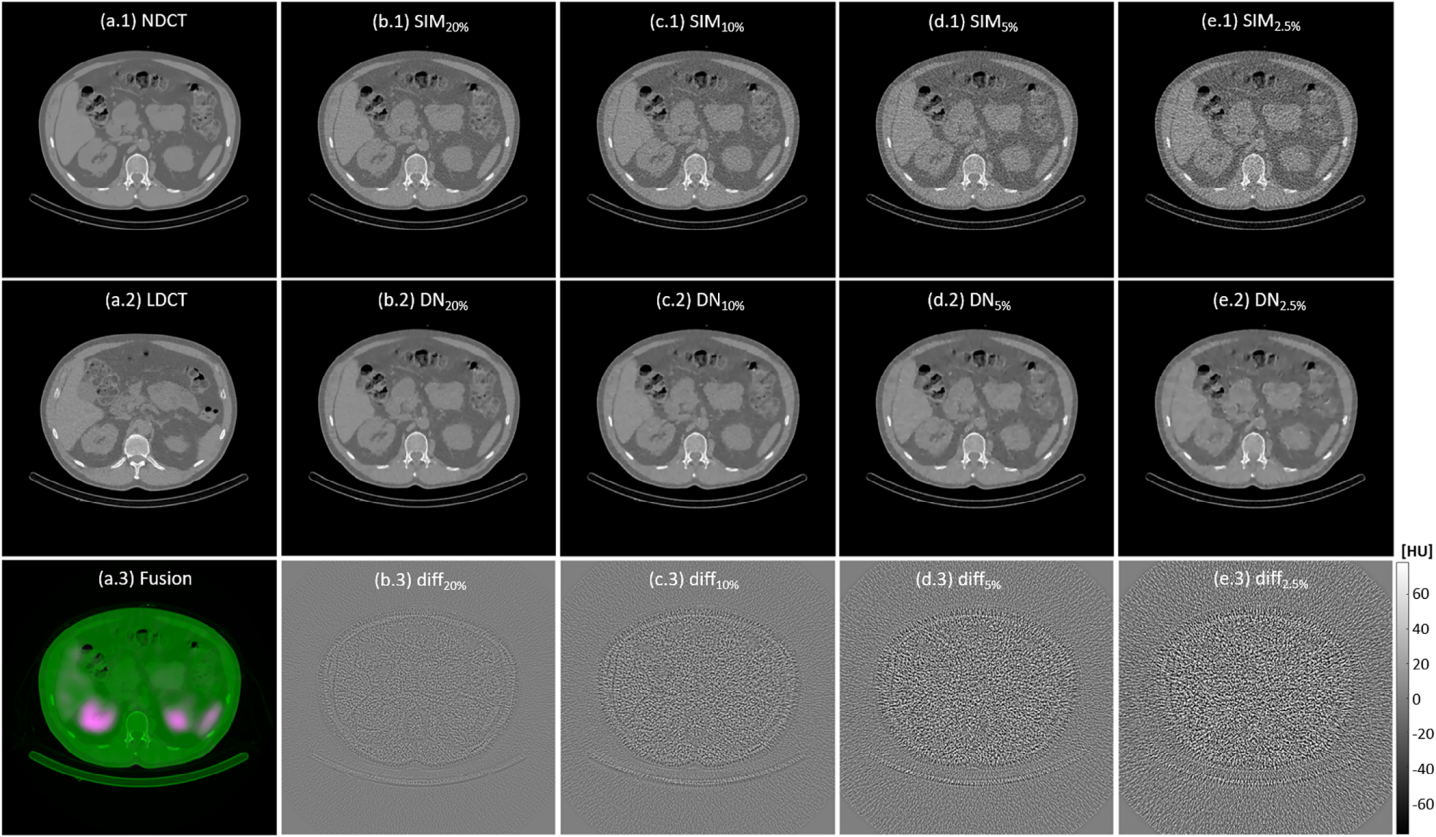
(a.1‐a.3) NDCT, LDCT, a fusion of SPECT (green) and NDCT (magenta); (b.1‐b.3) SIM_20%_, DN_20%_ and their difference (diff_20%_); (c.1‐c.3) SIM_10%_, DN_10%_ and their difference (diff_10%_); (d.1‐d.3) SIM_5%_, DN_5%_ and their difference (diff_5%_); (e.1‐e.3) SIM_2.5%_, DN_2.5%_ and their difference (diff_2.5%_).
